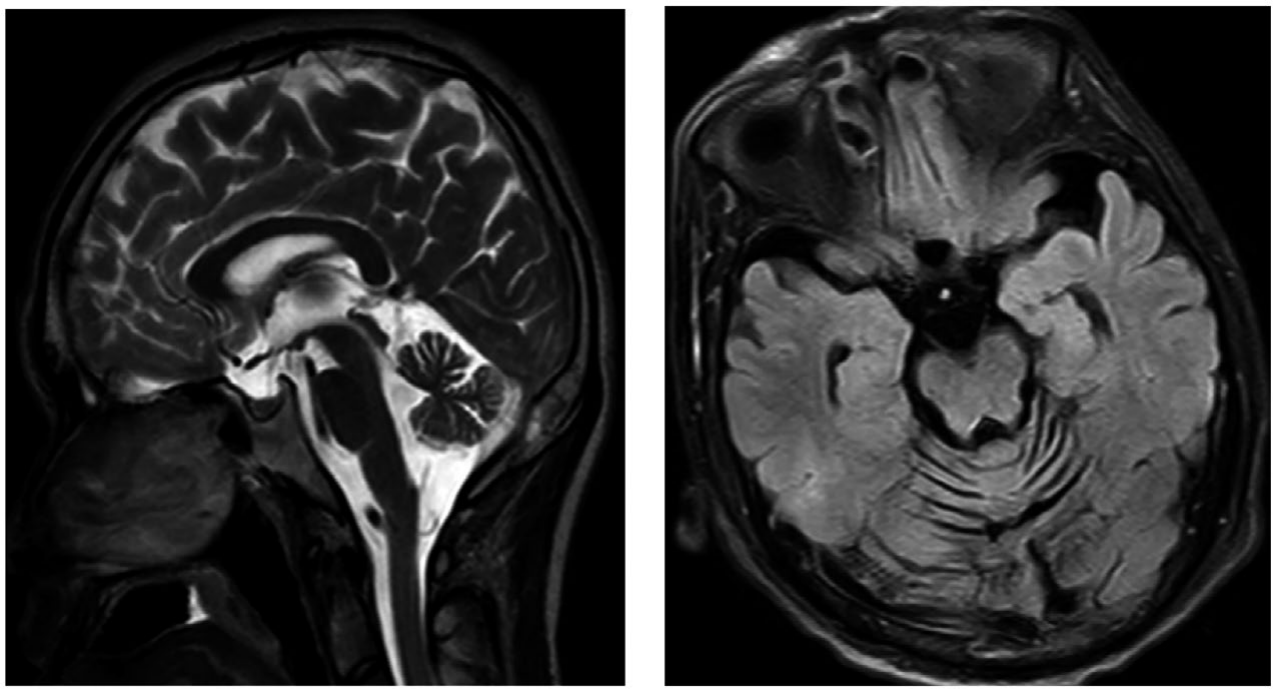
Brain MRI show cerebellar atrophy of the index patient(Ⅱ:2). Left: Sagittal scan, Right: axial scan
